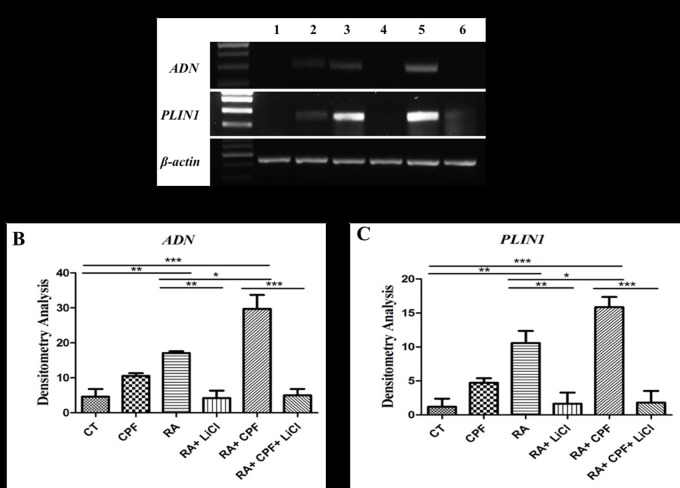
Adipogenic gene expression profile. (a) Electrophoretogram shows in lane (1) CT (2) 50 μM CPF (3) 2 μM RA (4) 2 μM RA+10 mM LiCl (5) 2 μM RA+50 μM CPF (6) 2 μM RA+50 μM CPF+10 mM LiCl. RT PCR results showing gene expression levels of *PLIN1* and *ADN*. *β-actin* was employed as housekeeping gene. Densitometry analysis depicting (b) 4, 9 and 13-fold increase in *PLIN1* gene expression of 50 μM CPF, 2 μM RA and 2 μM RA plus 50 μM CPF respectively, as compared to CT. A 2-fold increase was prevalent between RA and RA+CPF. (c)
